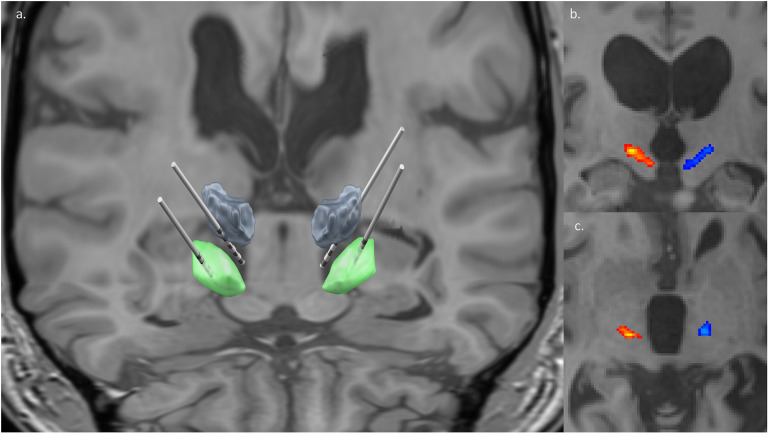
Lead and white matter tract reconstruction. (a) Four DBS [bilateral Vim/DRTT (gray), bilateral GPi (green)] leads reconstructed with patient’s preoperative T1 non-contrast MRI as backdrop and DISTAL atlas segmentations of deep brain nuclei. Two distal contacts of Vim leads can be seen intentionally deep to the nucleus, targeting white matter fiber tracts. Lead visualization generated in Lead-DBS (Horn et al., 2019). (b,c) The right (red) and left (blue) DRTT (dentato-rubro-thalamic tract) are overlaid onto the patient’s T1 non-contrast MRI. The coronal slice (b) showcases the tracking course of the DRTT, while the axial slice (c) captures the DRTT along the AC/PC plane. Tractography images generated in FSL.
